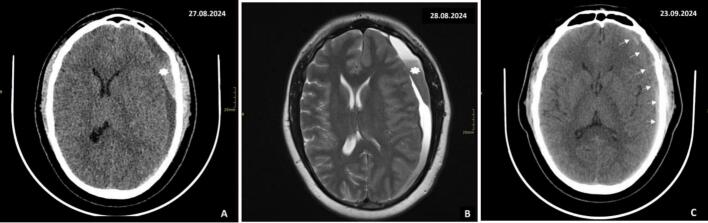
A. Plain CT of the brain, 2 weeks after initial treatment, demonstrated new mixed hyper and hypodense collections within the left subdural space (star) measuring 14.5 mm at the thickest point. It was causing a significant mass effect with a midline shift of about 9.2 mm. There was diffuse effacement of sulci at the left hemisphere. B. Plain MRI of the brain, 2 weeks after initial treatment, demonstrated left frontoparietal-temporal-occipital and temporal subdural collection (star) measuring 13 mm at the thickest point, causing mass effect onto the adjacent sulci with significant midline shift(6 mm). There was Minimal subfalcine herniation. C. Plain CT of the brain, 4 weeks after initial treatment, demonstrated a hyperdense subdural collection in the left subdural space (arrows) with a hypodensity in the non-dependent part measuring about 8.6 mm on the thickest part. There was a mass effect and an ipsilateral sulcal effacement with a mild midline shift of about 5 mm.
